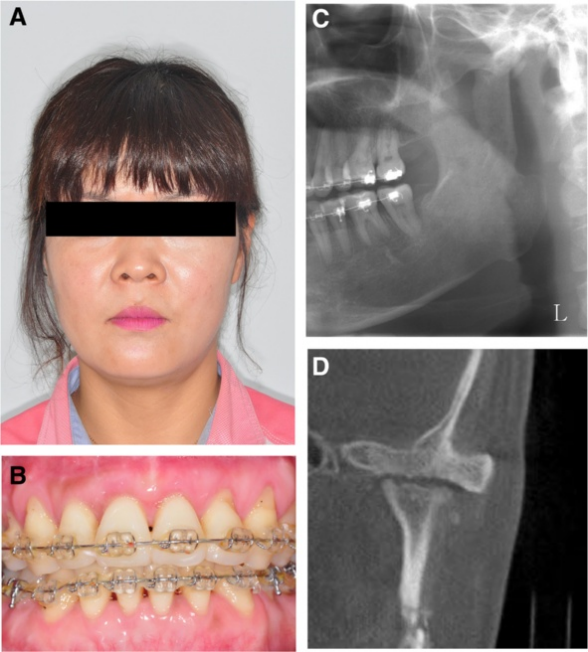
Post-operative patient information. (A) The patient showed no facial asymmetry. (B) The midline of the lower teeth was corrected. (C) Panoramic radiograph shows no signs of recurrence. (D) Cone-beam computed tomography image. The condyle was in a bone remodeling state.
